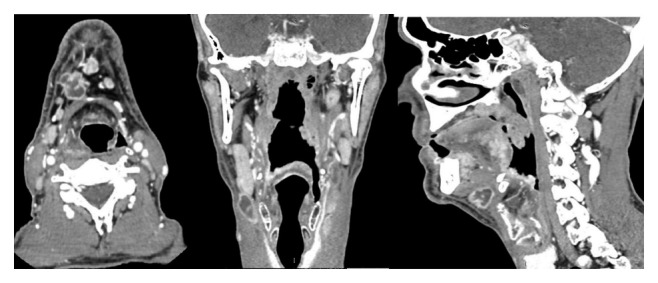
Computed tomography cuts showing lymph node enlargement as a hypodense and heterogeneous image in the right submandibular region.
